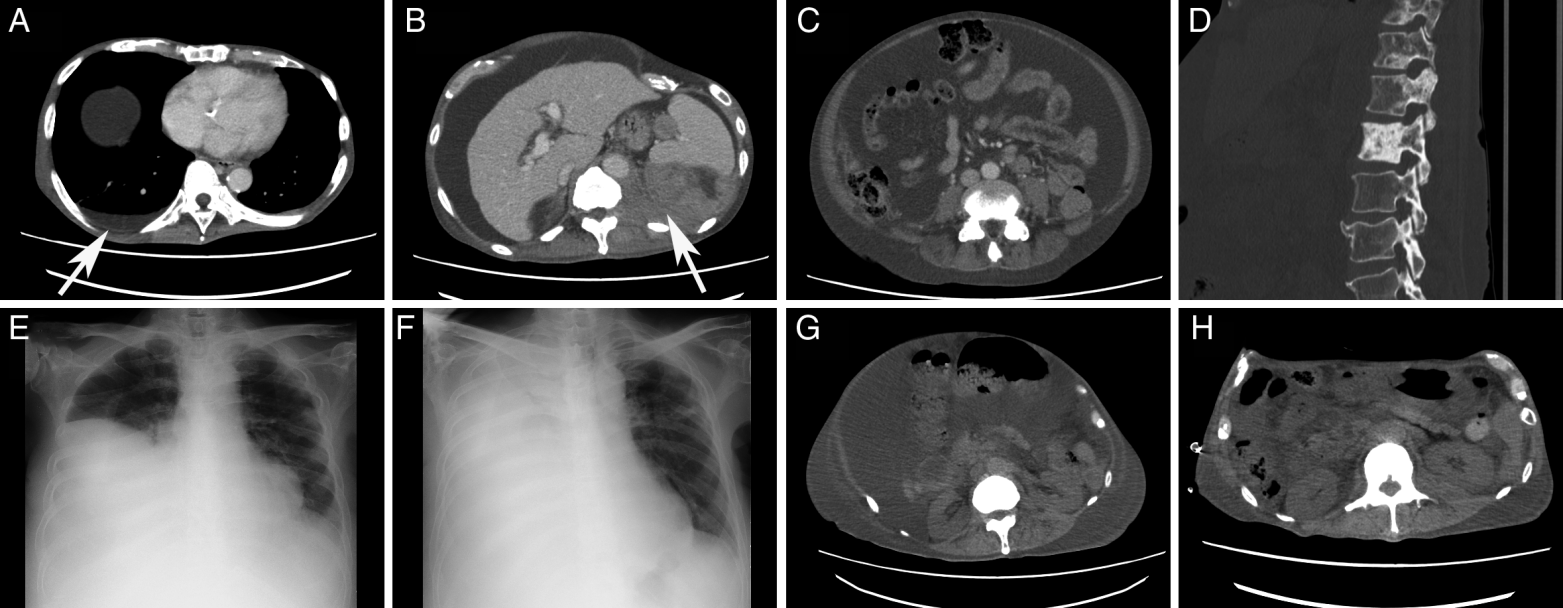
(A) Abdominal computed tomography (CT) scan shows pleural effusion (white arrow); (B) abdominal CT scan shows recurrent thymoma (white arrow); (C) abdominal CT scan shows massive ascites; (D) CT scan shows multiple bone metastases; (E) chest X‐rays show before thoracentesis and (F) 2 h after thoracentesis; and (G) abdominal CT scans show before thoracentesis and (H) after thoracotomy tube insertion. (A, B, C, G, H) Images obtained with mediastinal window settings. (D) An image obtained with bone settings.
